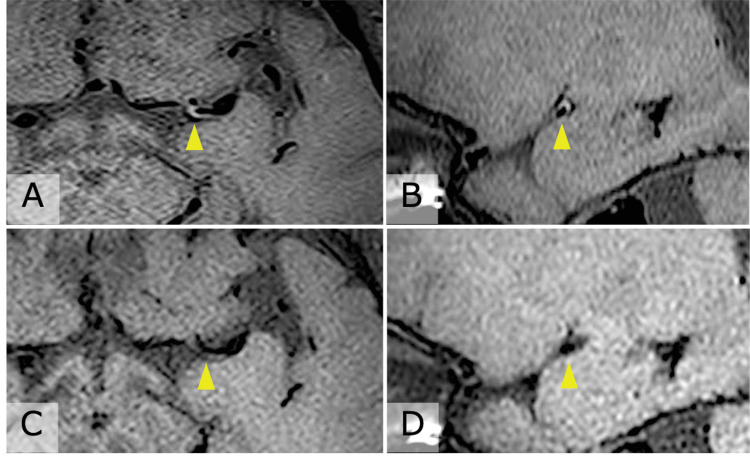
MRI black-blood sequences before and after corticosteroid therapy. (A, B) Pre-treatment images showing concentric enhancement of the left middle cerebral artery wall (yellow arrowheads). (C, D) Post-treatment images after intravenous methylprednisolone pulse therapy demonstrating resolution of vessel wall enhancement (yellow arrowheads).
